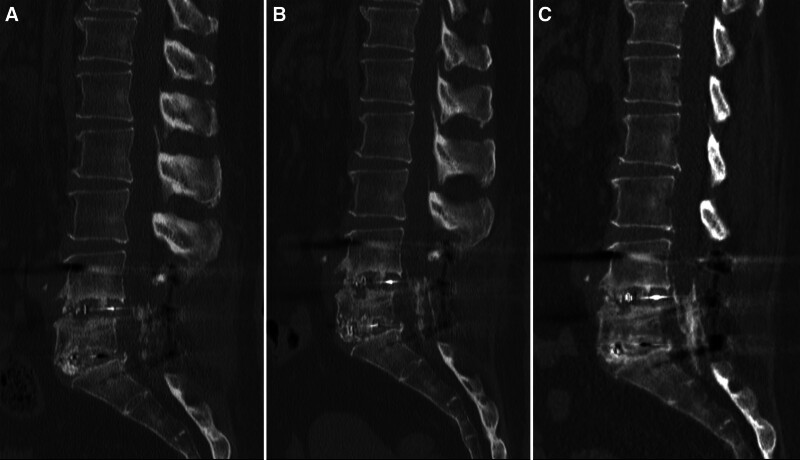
Sagittal CT images of ossification progression: (A) identifies the presence of a small amount of filamentous ossification within the spinal canal on postoperative month 10. (B) reveals an increase in filamentous ossification compared to previous scans, forming a reticular pattern on postoperative month 14. (C) shows progressive ossification, with a denser and more extensive reticular pattern on postoperative month 25. CT = computed tomography.
